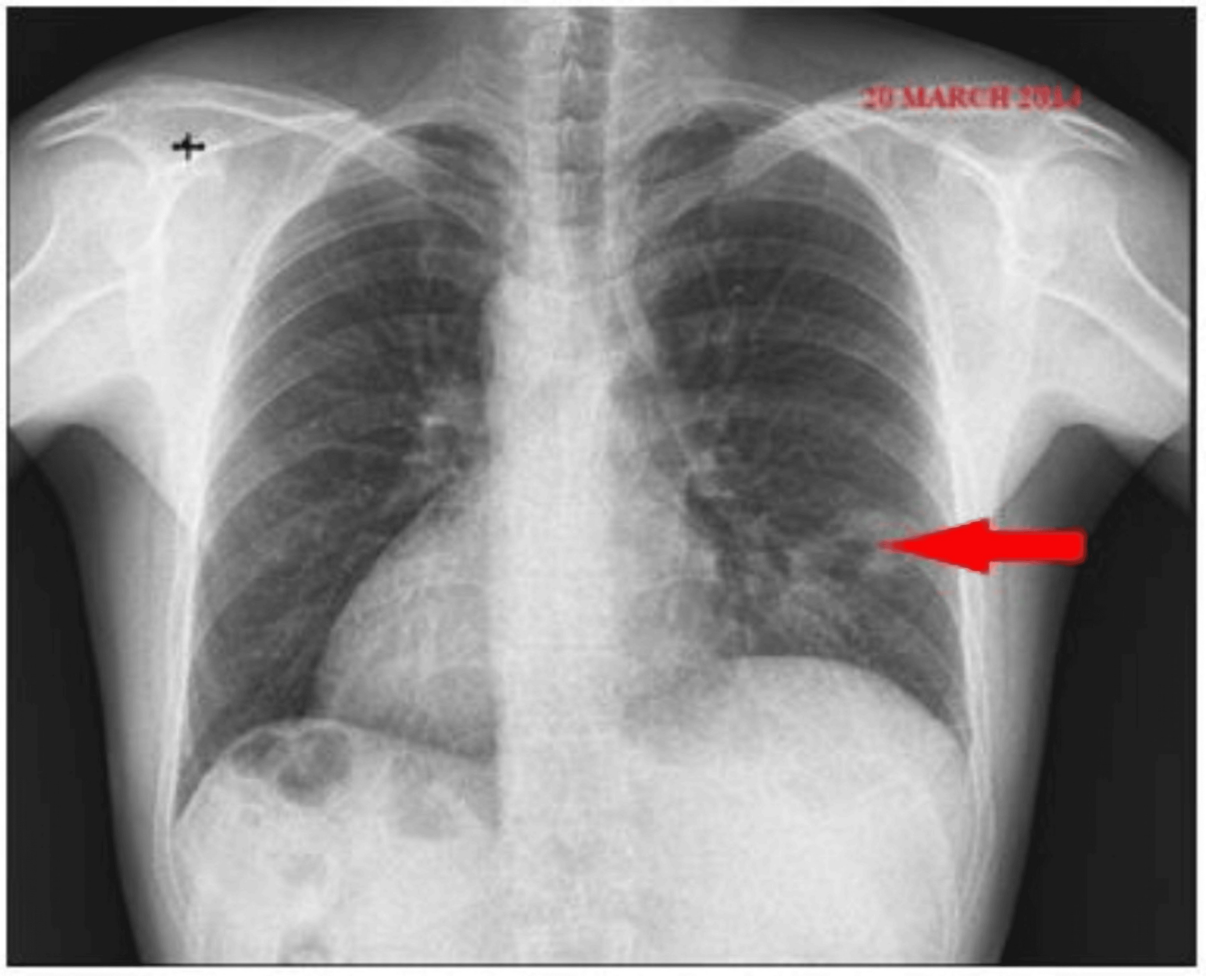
Chest X-ray showing persistence of the left paracardiac pulmonary infiltrate
